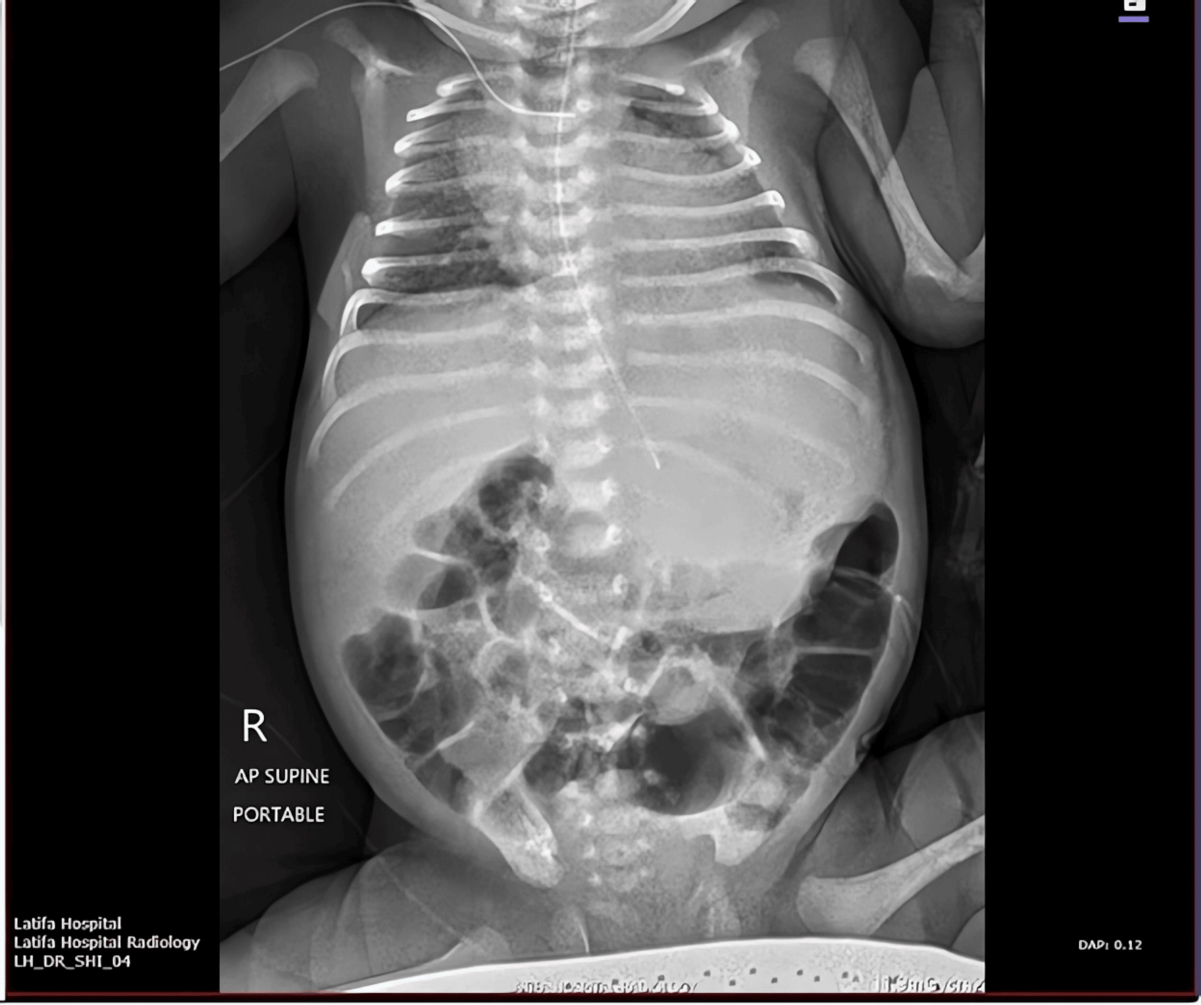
Day 11 radiograph demonstrating progressive enlargement of calcified epigastric mass Abdominal radiograph on day 11 demonstrating progressive enlargement of the epigastric mass and increasing calcific density within the lesion, with no evidence of bowel obstruction or free air.
